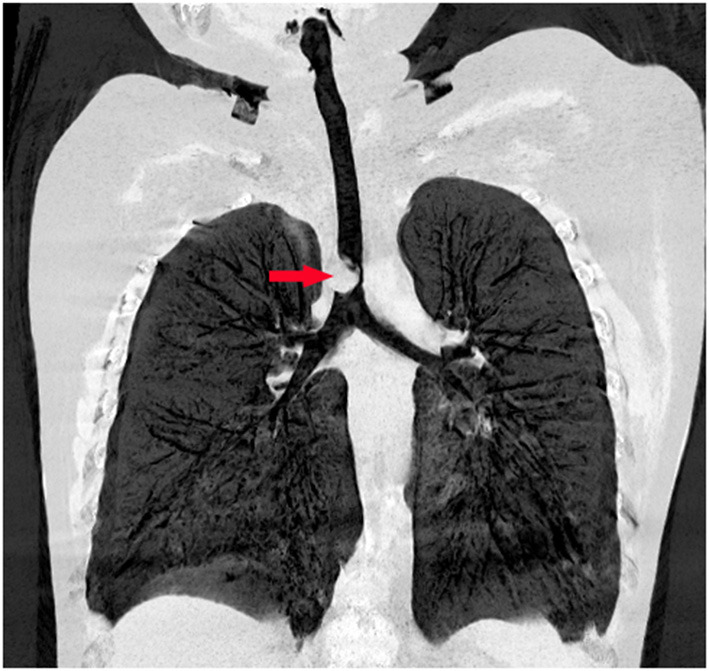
Preoperative chest computed tomography reveals an endotracheal tumor nearly totally obstructing the tracheal lumen.
